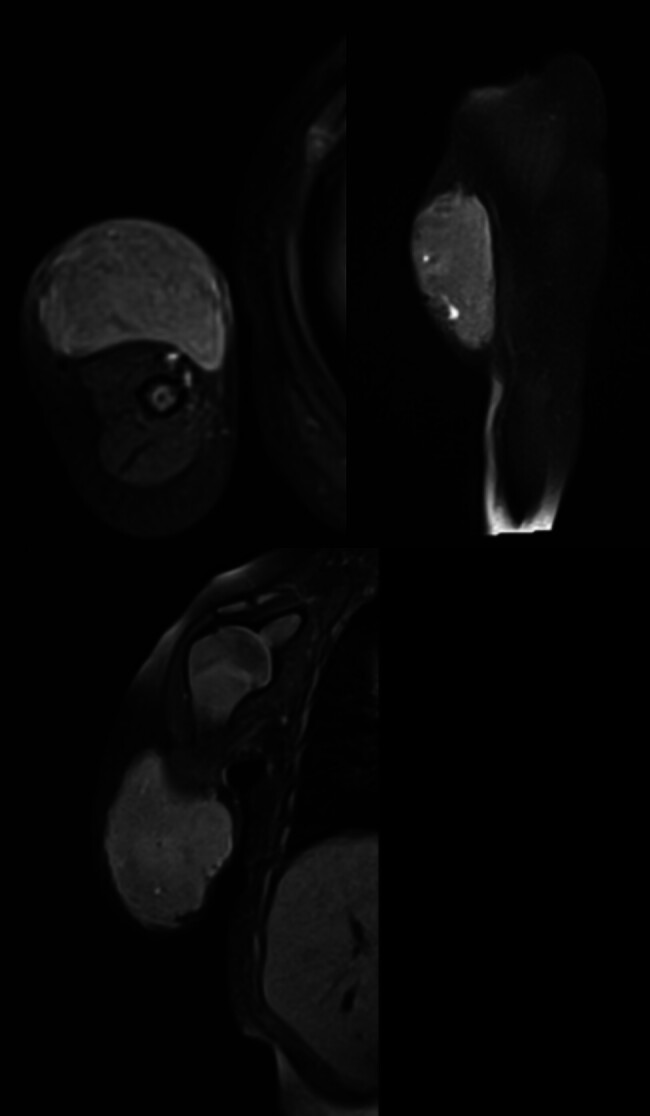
Axial, sagittal, and coronal fat-suppressed proton density MR images of the right arm showing a well-defined subcutaneous fusiform lesion with heterogeneous signal intensity. No frank intramuscular extension or cortical invasion is evident.
